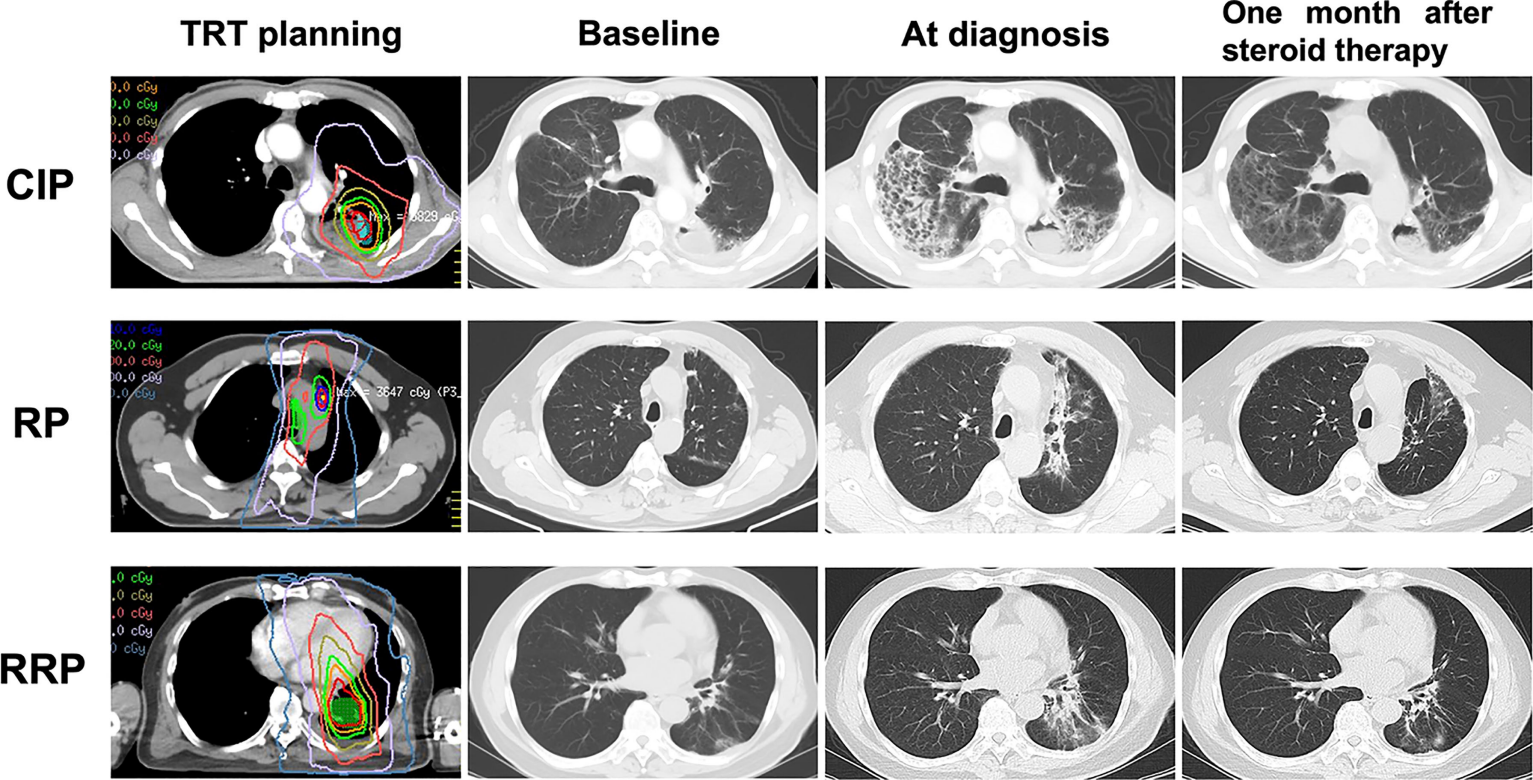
Distinctive CT changes of CIP, RP and RRP at baseline, at diagnosis and one month after steroid therapy. CIP, checkpoint inhibitor pneumonitis; RP, radiation pneumonitis; RRP, radiation recall pneumonitis; TRT, thoracic radiotherapy.
